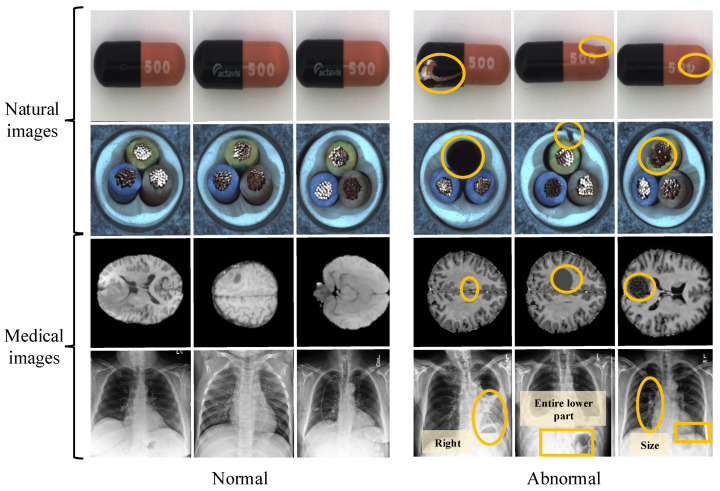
The first two rows represent natural images, and the yellow circles highlight multiple types of defects: sealing defects, dents, and scratches in the first row; hollow, insulation layer defects and conductor deviation in the second row. The third row contains normal and abnormal (inflammatory, lesions and bleeding) MRI medical images, and the fourth row shows the normal and abnormal (pneumonia, effusion, and nodule) medical images from the ZhangLab dataset. Unlike natural images, anomaly detection in smart health medical images focuses more on the individual shape and location of the target for accurate diagnosis.
